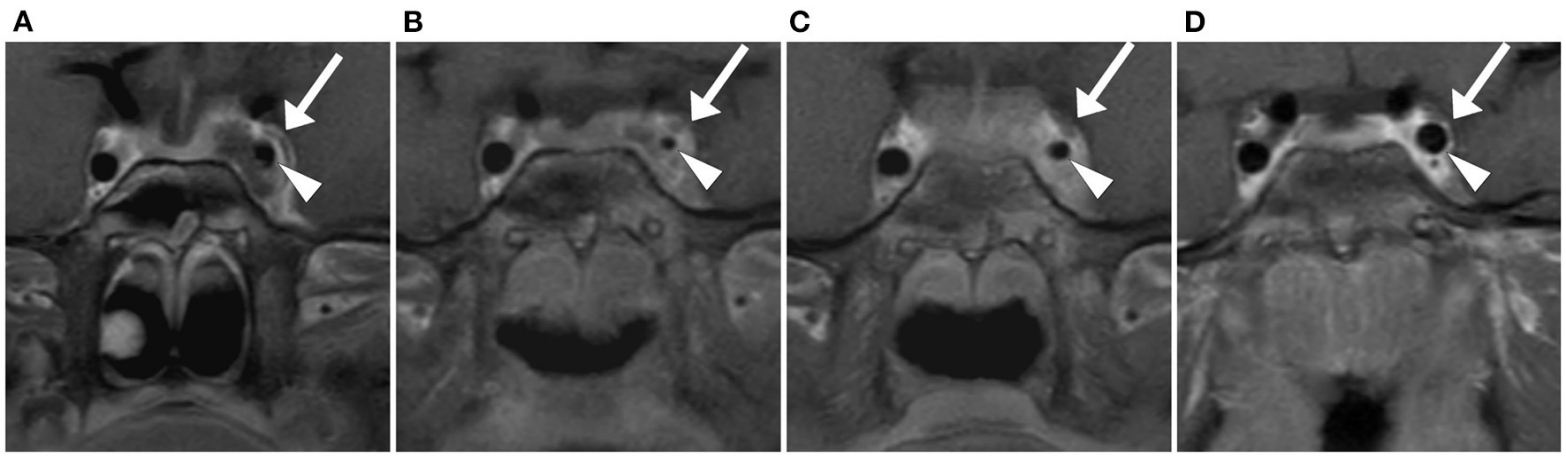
Coronal view T1 DIXON FS post gadolinium, slice thickness 3 mm. Arrow: Cavernous sinus, Triangle: Pars cavernosa of the internal carotid artery. Initial MRI: Thrombosis of the cavernous sinus and involvement of the internal carotid artery with hyperemia of the vasa vasorum (A). MRI 7 days post-admission: Increasing inflammation of the internal carotid artery with further reduction in lumen size (B). MRI 14 days post-admission: Improvement of internal carotid artery involvement with a partial recovery in size (C). MRI 3 months post-admission: Complete recanalization of the left cavernous sinus and normal size of the left internal carotid artery (D).
